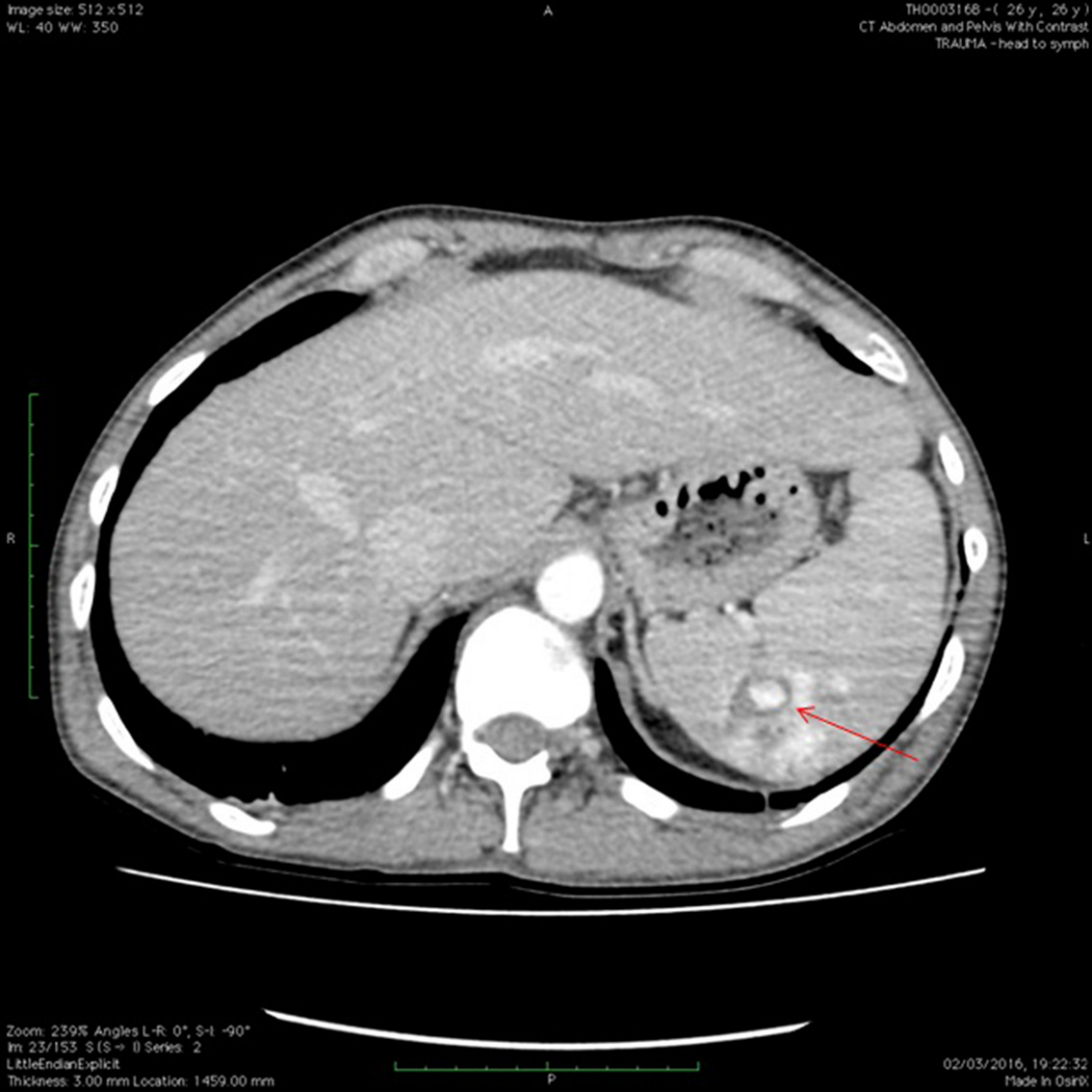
Axial CT scan of abdomen/pelvis with contrast at 70s. Active haemorrhage now visible (red arrow) within the spleen indicating acute injury.
